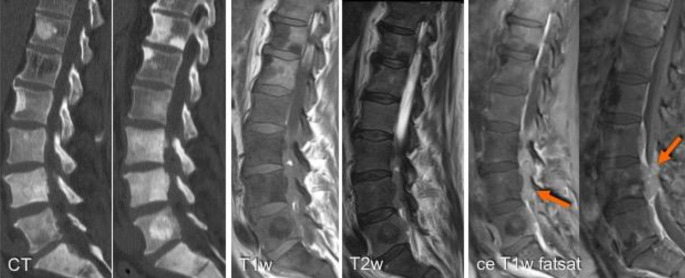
Computed tomography (*CT*) and multiparametric magnetic resonance imaging including T1-, T2- as well as pre- and postcontrast T1-weighted (*T1w*) fat-saturated (*fatsat*) imaging. Note the variable appearances of the lesions on T1, variable enhancement patterns, and superior demonstration of the soft tissue component of the metastatic bone disease at L3 and L4, extending into the spinal canal and posterior elements (*arrows*)
